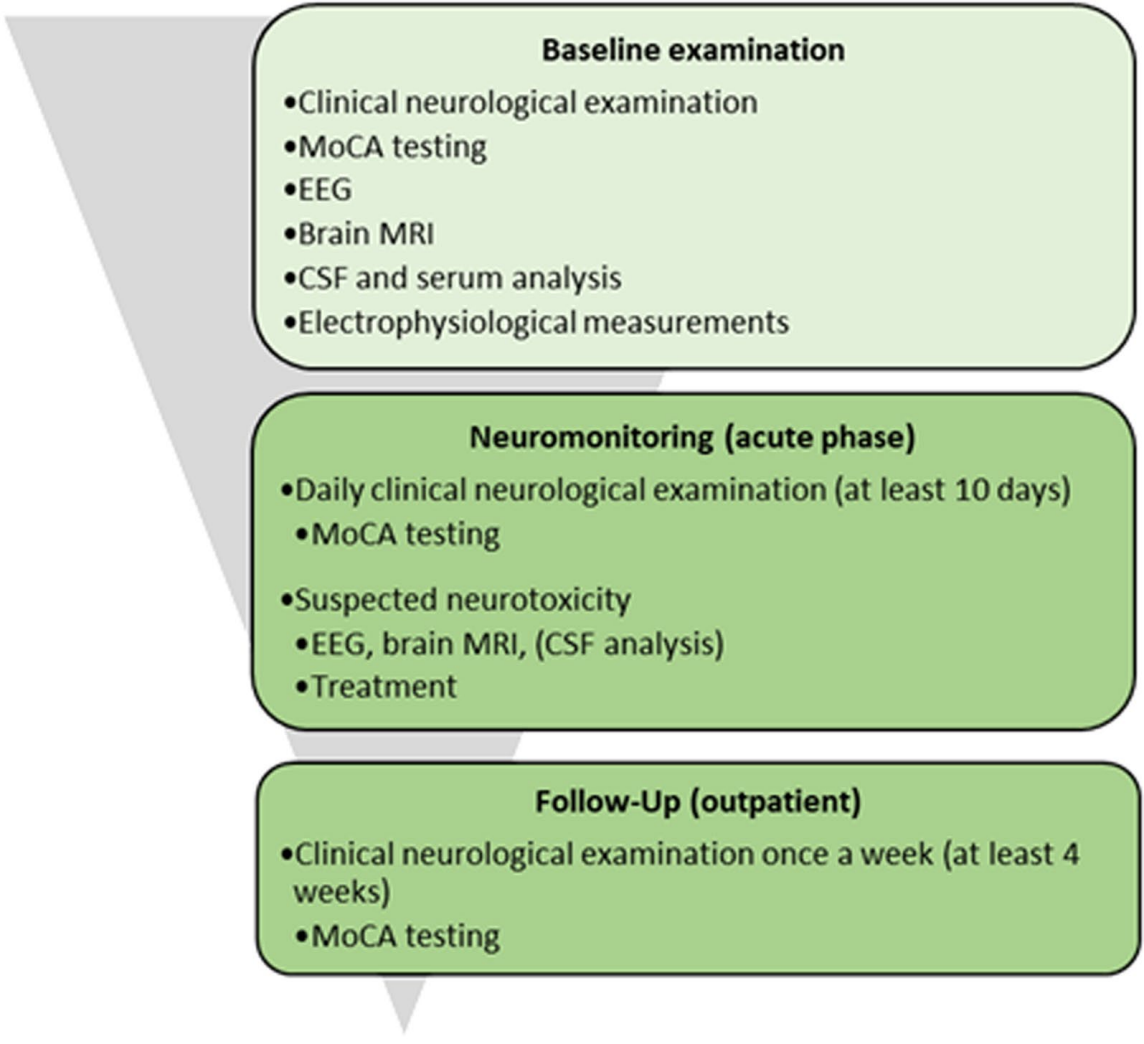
Hannover cohort neurological examination and monitoring before, during and after CAR T cell treatment. CSF: cerebrospinal fluid; EEG: electroencephalography; MoCA: Montreal Cognitive Assessment; MRI: magnetic resonance imaging
